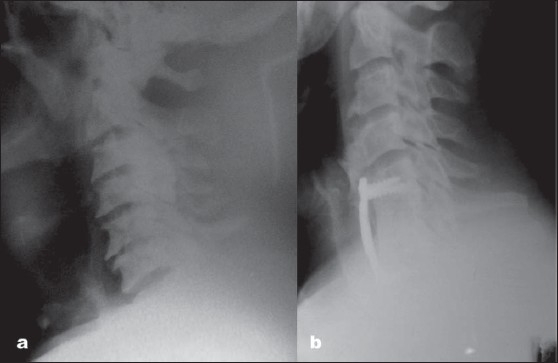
(a) X-ray cervical spine (lateral view) showing cervical spondylotic myelopathy in 43-year-old man with acute onset and rapid progression of symptoms. His preoperative Nurick's grade was 5 and mJOA score of 6; (b) Postoperative X-ray cervical spine (lateral view) showing corpectomy and anterior plate. His Nurick's grade was 1 and mJOA score was 17, with a recovery rate of 91.66%
